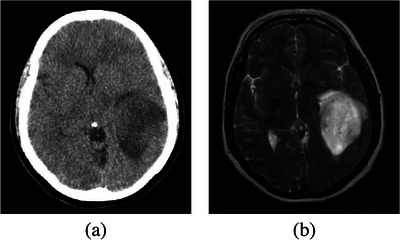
A sample of multimodal images for the registration from our local database. (a) Brain CT; (b) *T*
